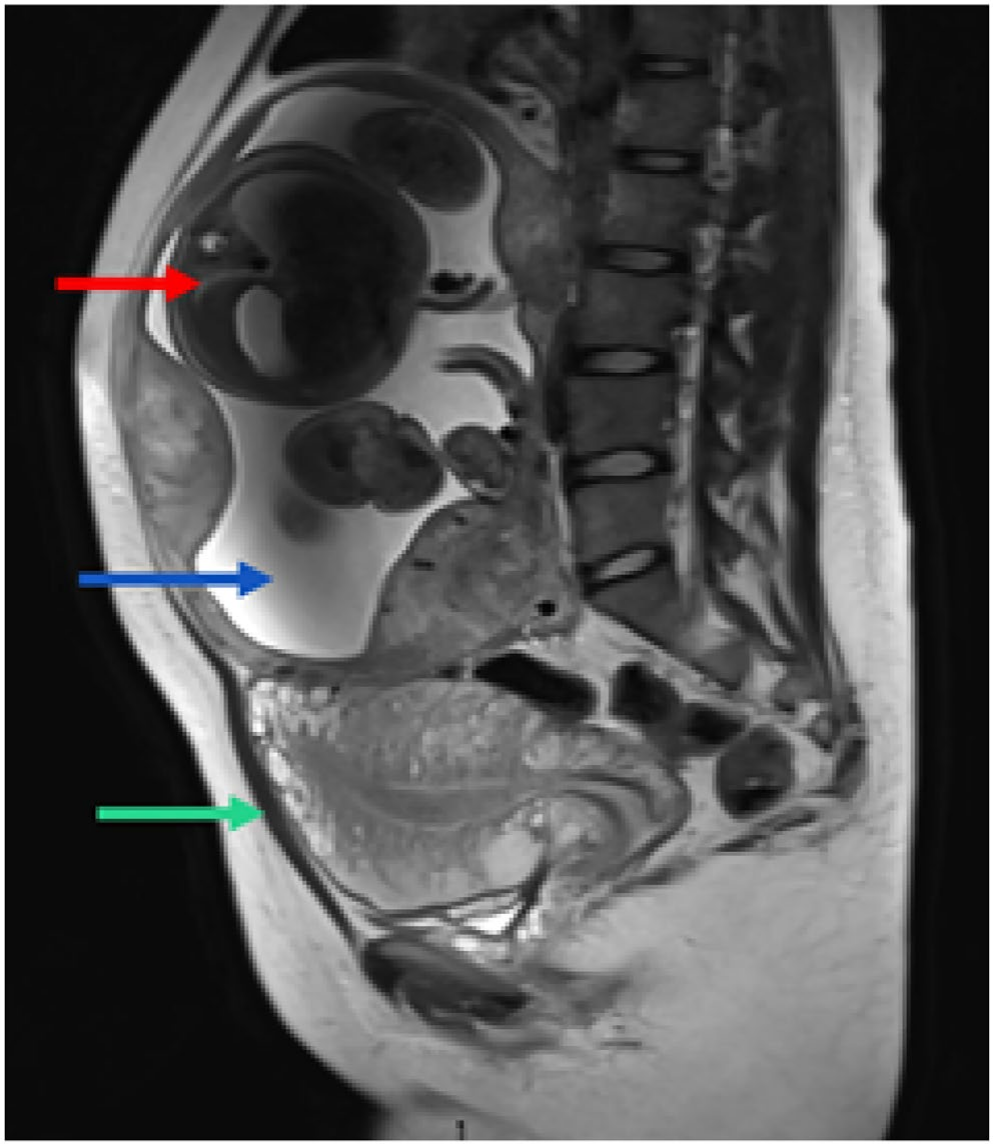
MRI sagittal view. MRI showing an abdominal pregnancy (with gestational age of 32 weeks as per LMP) with empty uterus (green arrow) normally visualized in the pelvis, without any definite communication of the fetal sac with the uterus/cervix with the fetus (red arrow) and placenta (blue arrow) implanted in the abdomen. Sagittal view shows multilobulated placenta located in the anterior and posterior segments.
